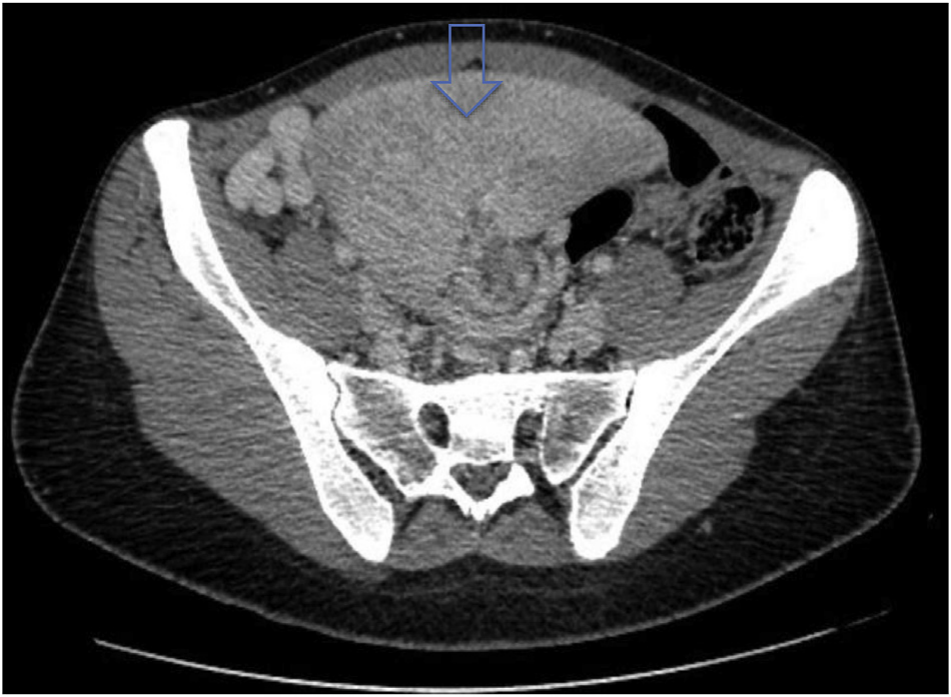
Contrast-enhanced axial CT image demonstrates the spleen in its pelvic position and the so called “whirl sign” of the splenic pedicle.
